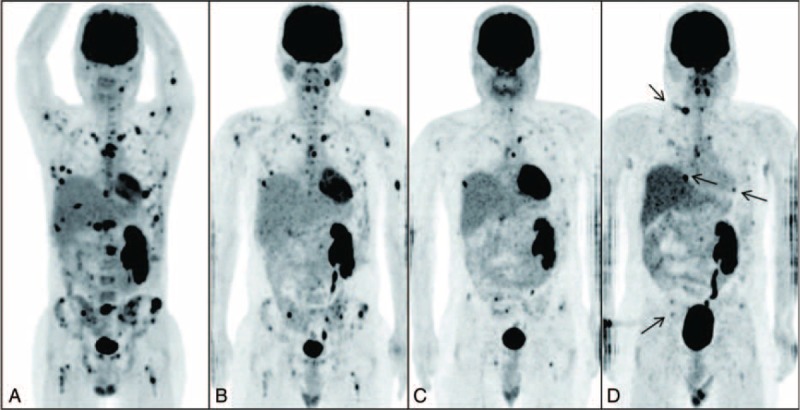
The serial positron emission tomography-computed tomography scans (A) before and (B) after thalidomide/chemotherapy; (C) day +65 and (D) day +300 posttransplant show decreasing [18F]fluorodeoxyglucose (FDG) uptake within lymph nodes in the upper abdominal, paraaortic regions, and also in skull, clivus, left mandible, cervical, thoracic and lumbar spines, pelvic bones, sacrum, sternum, ribs, clavicles, right scapula, bilateral humeri, and bilateral femora. Arrows point at the newly increased FDG-avid lesions in the upper back, right lung, and several bones on day +300 (D) compared with those on day +65 (C) posttransplant.
